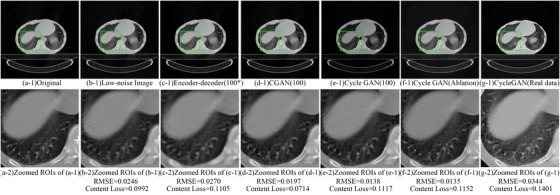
Example of low‐dose CT denoising. (a‐1) The original full dose CT image; (b‐1) Low‐noise image; (c‐1) image denoised by EDN (*Training at 100 epochs); (d‐1) image denoised by CGAN; (e‐1) image denoised by a cycle GAN; (f‐1) image denoised by a cycle GAN (ablation study); (g‐1) image denoised by cycle GAN trained on real data; (a‐2) to (g‐2) zoomed ROIs for (a‐1) to (g‐1).
